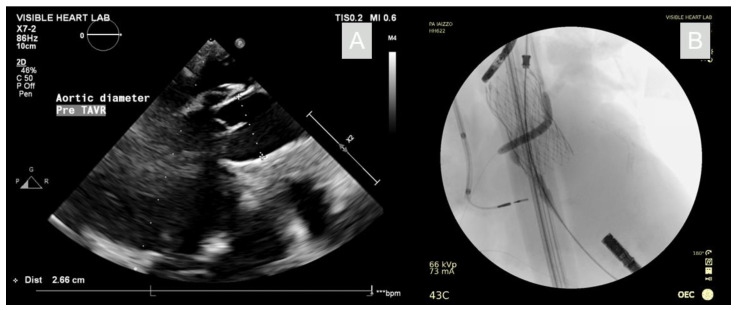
Echocardiography with aortic diameter measurement for prosthesis sizing (A). Fluoroscopy of the deployed TAVR and chimney stent with deployment balloon inflated (B).
